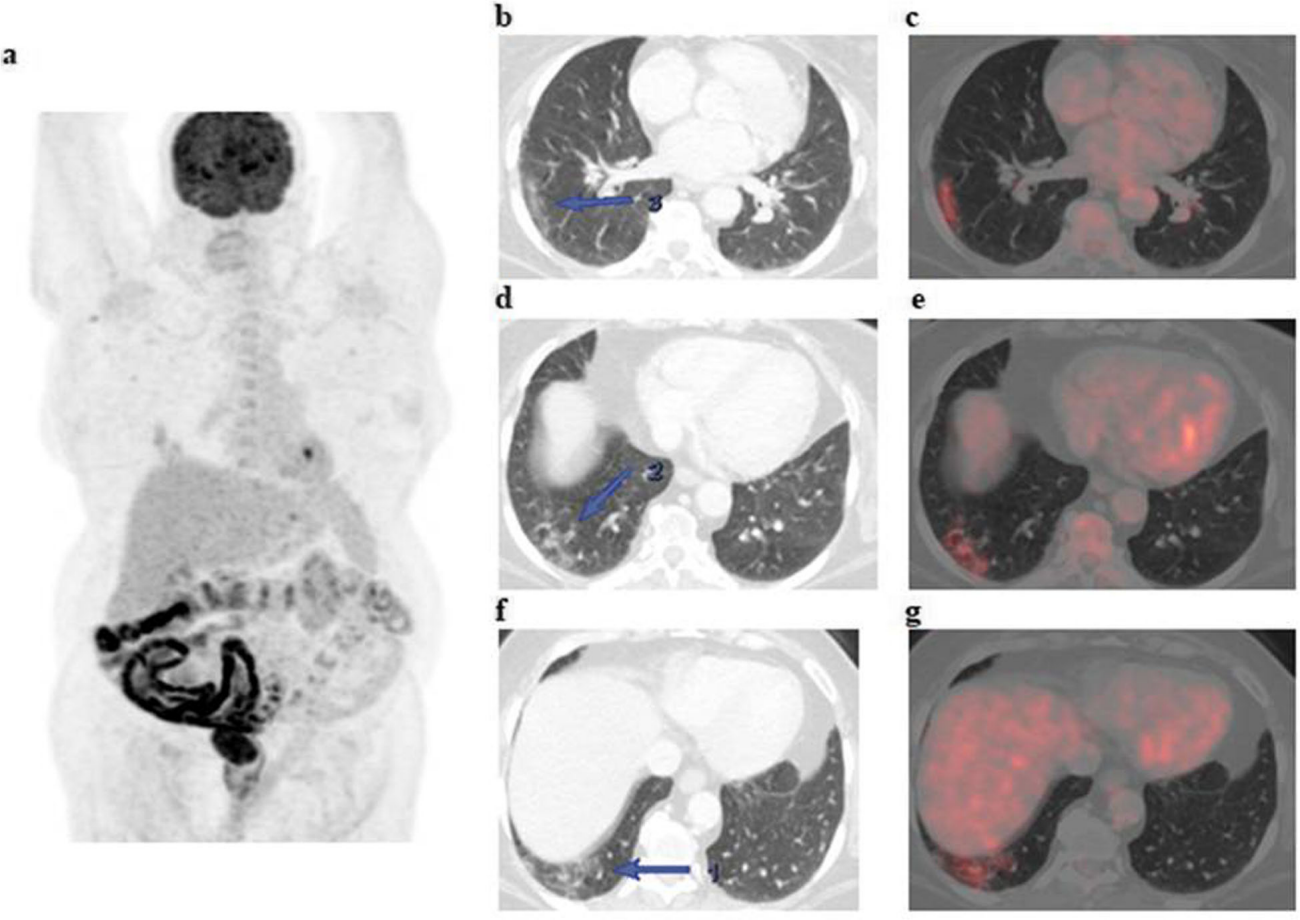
A 75-year-old female patient, with a history of lymphoma, received chemotherapy and referred for follow-up PET/CT evaluation. a The whole-body MIP image shows mildly active areas at the right lung lower zone. Axial lung window CT images (b, d, f) revealed few unilateral subpleural variable-sized ill-defined ground-glass opacities at the right lower lung lobe that show mild FDG uptake in corresponding fused PET/CT images (c, e, g) achieving up to 4.5 SUVmax
